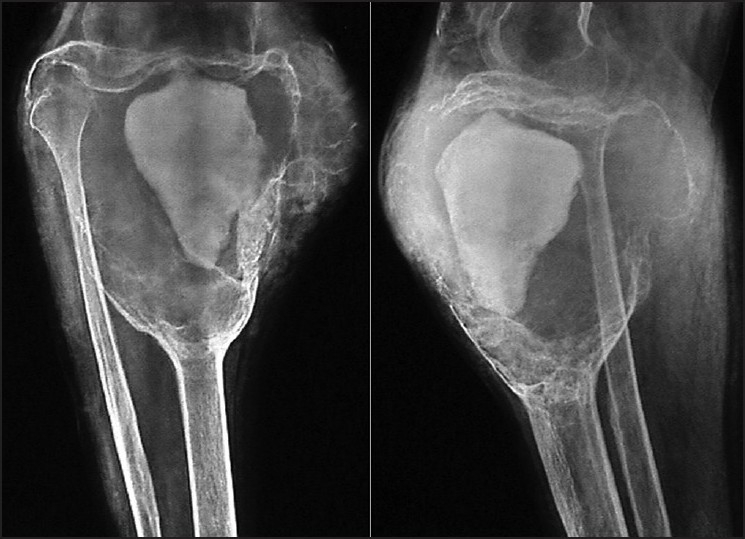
X-rays (anteroposterior, lateral views) of proximal both bones leg with knee joint showing recurrence at 28 months after initial refusal for surgery
